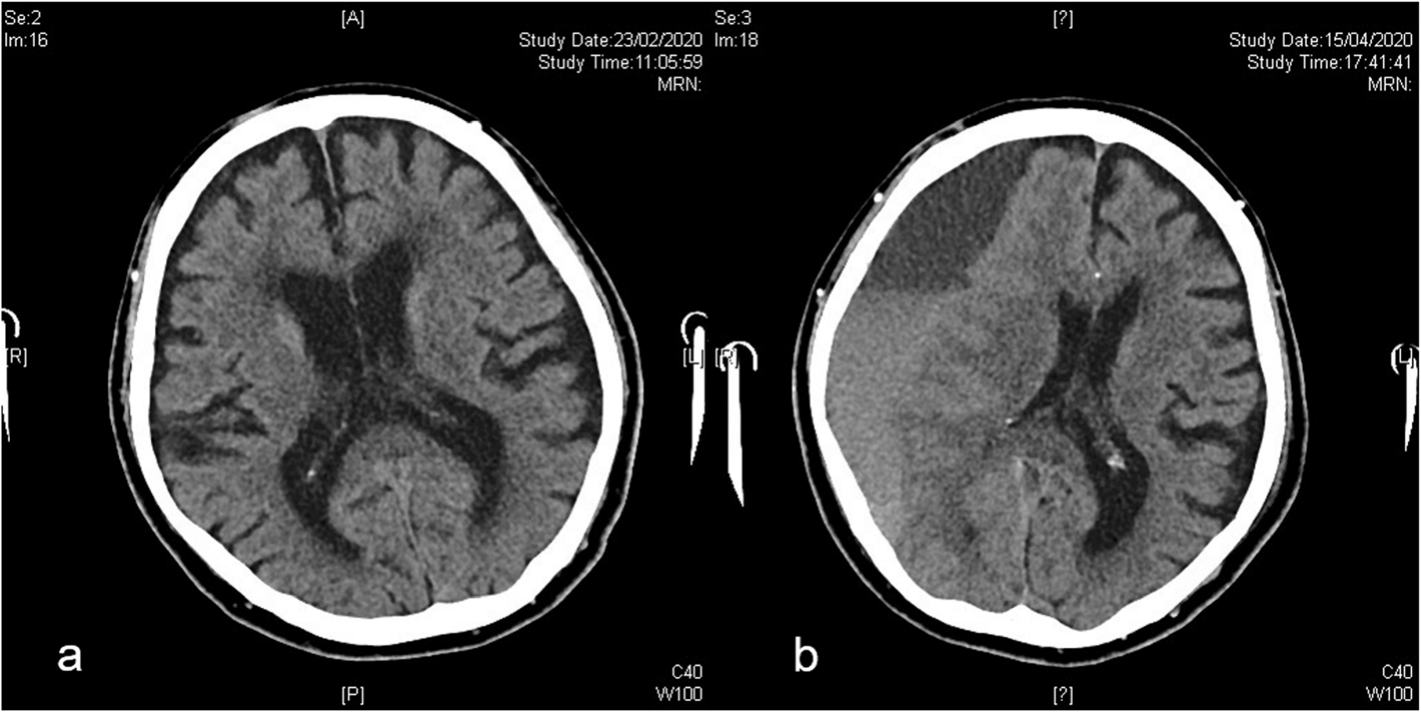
a CT brain scan of a 97-year-old gentleman at the time of injury. He had slipped and had a fall on level ground with a minor head injury. CT scan initially showed no intracranial hemorrhage and he was discharged with medical advice. Subsequently, he had progressively worsening left-sided weakness and clumsiness but did not go to the hospital immediately in view of the pandemic. b He attended the hospital 7 weeks and 3 days after his initial minor head injury. The new CT showed a newly diagnosed right-sided chronic subdural hematoma with mass effect and midline shift. Despite the delay in presentation, he had a good recovery after emergency burr hole drainage
